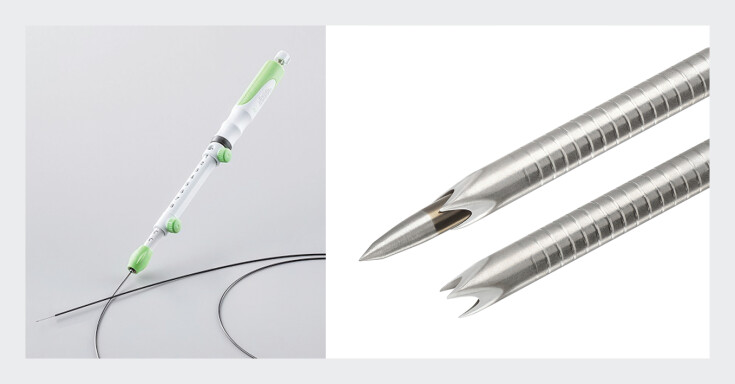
A novel Franseen needle (Acquire-S) has a sharp and tapered stylet for ease of puncture when performing endoscopic ultrasound-guided tissue acquisition (EUS-TA). The tapered stylet can be used in the advanced or retracted position based on the physician's preference. Source: Boston Scientific Corporation.
